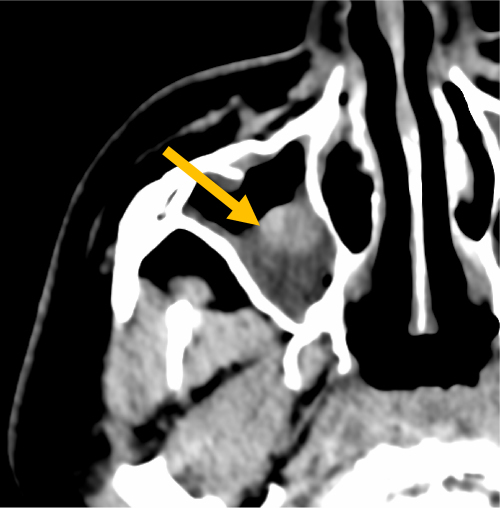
Computed Tomography of the Paranasal Sinuses (Axial Plane, Soft-tissue Window). Heterogeneous content with hyperdense areas is seen in the right maxillary sinus (arrow). Intraoperative data for this patient revealed a polyp and no evidence of a fungal process. Hence, hyperdense inclusions in the structure, which are considered to be pathognomonic for a fungal character, may indicate an unchanged polyp due to its high protein content.
